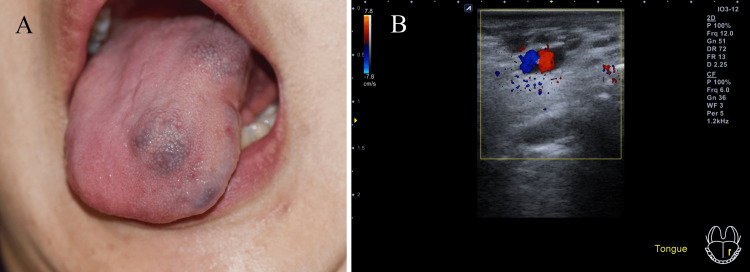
Vascular lesion on the anterior region of the tongue before sclerotherapy (A) Clinical appearance; (B) Ultrasonography doppler image
